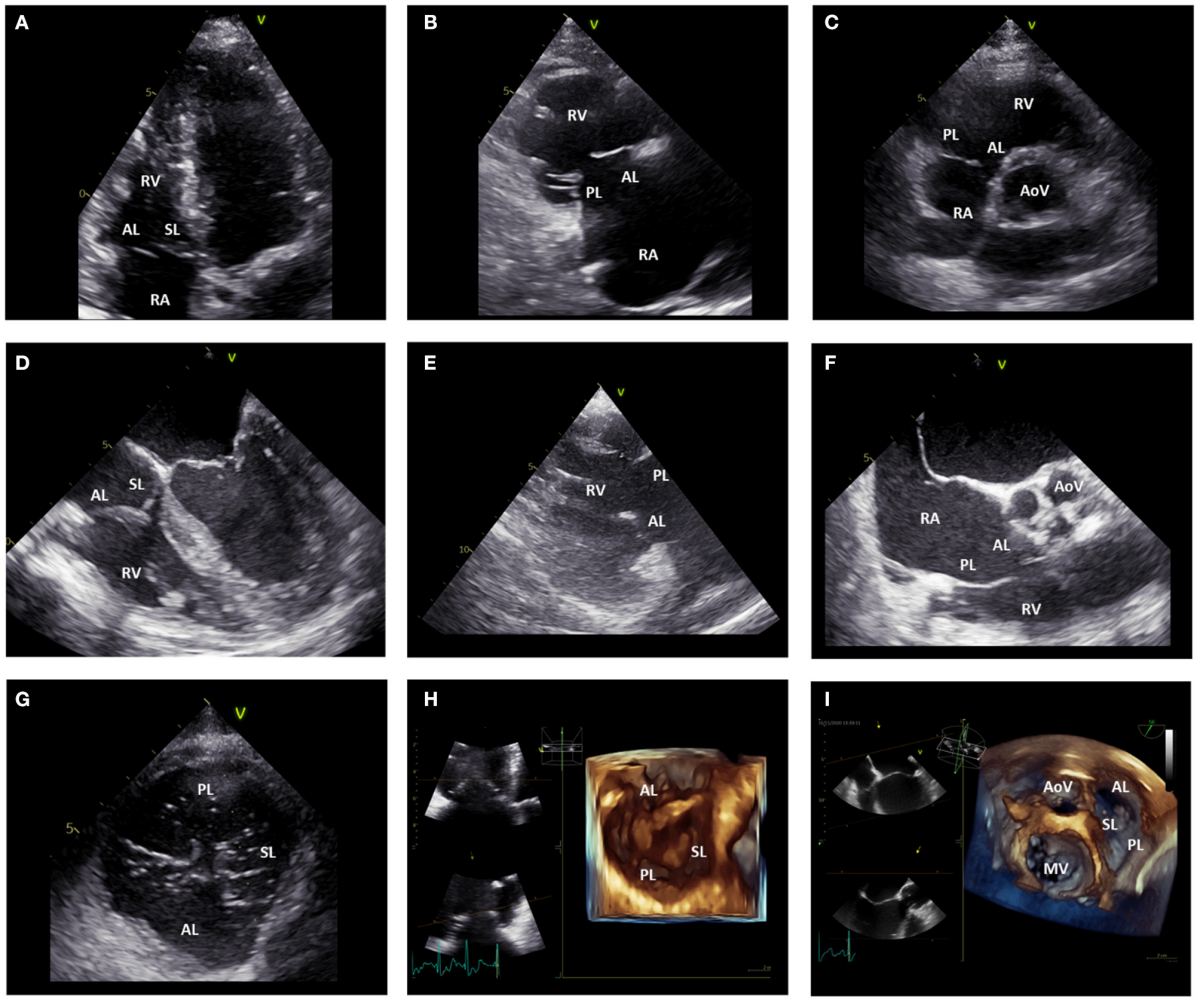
Echocardiographic assessment of the tricuspid valve. (A) displays the apical four-chamber view where the anterior and septal leaflet of the TV can be visualized. (B) shows the parasternal long-axis view of the RV where the anterior and posterior leaflet of the TV can be imaged. In the parasternal short-axis view (C), the posterior and anterior leaflet of the TV can be imaged. (D) shows the mid-esophageal four-chamber view where the anterior and septal leaflet of the TV can be observed. (E) displays the transgastric RV inflow view where the posterior and anterior leaflet of the TV can be imaged. From the mid-esophageal RV inflow-outflow view (F) the anterior and posterior leaflet of the TV can be imaged. From the transgastric TV short-axis view (G), all the TV leaflets can be simultaneously visualized. Similarly, 3D images of the TV acquired from the apical (H) and mid-esophageal (I) four-chamber view can show all the TV leaflets simultaneously. AL, anterior leaflet; AoV, aortic valve; MV, mitral valve; PL, posterior leaflet; RA, right atrium; RV, right ventricle; SL, septal leaflet; TV, tricuspid valve.
